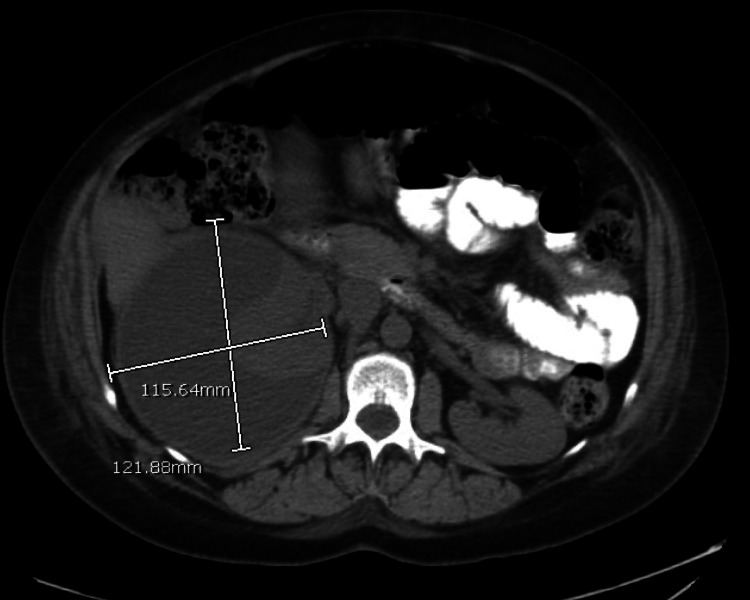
Axial CT showing dimensions of the renal abscess
